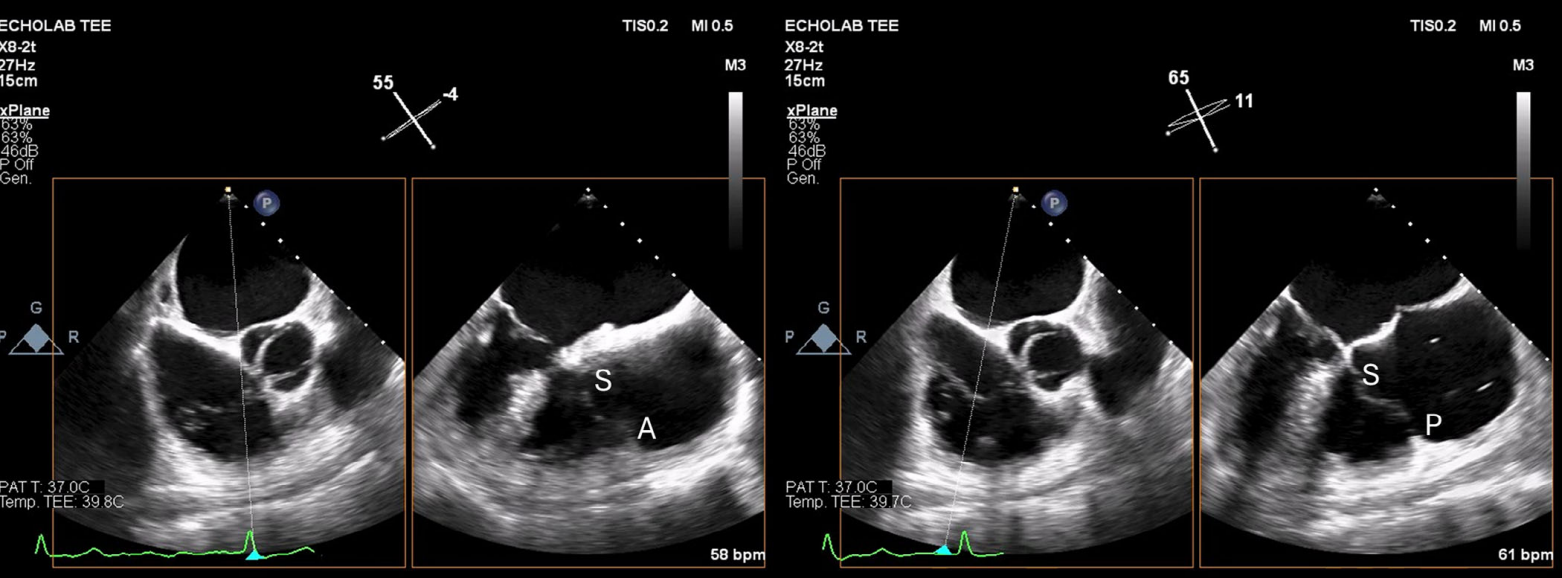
TOE mid‐esophageal 60° RV inflow‐outflow view with simultaneous biplanar imaging. On the left image simultaneous biplanar imaging is positioned to obtain a 90° view for the S and the A. On the right image the simultaneous biplanar imaging is positioned to obtain a 90° view for the S and the P. A indicates anterior leaflet; P, posterior leaflet; RV, right ventricle; S, septal leaflet; TOE, transesophageal echocardiography.
